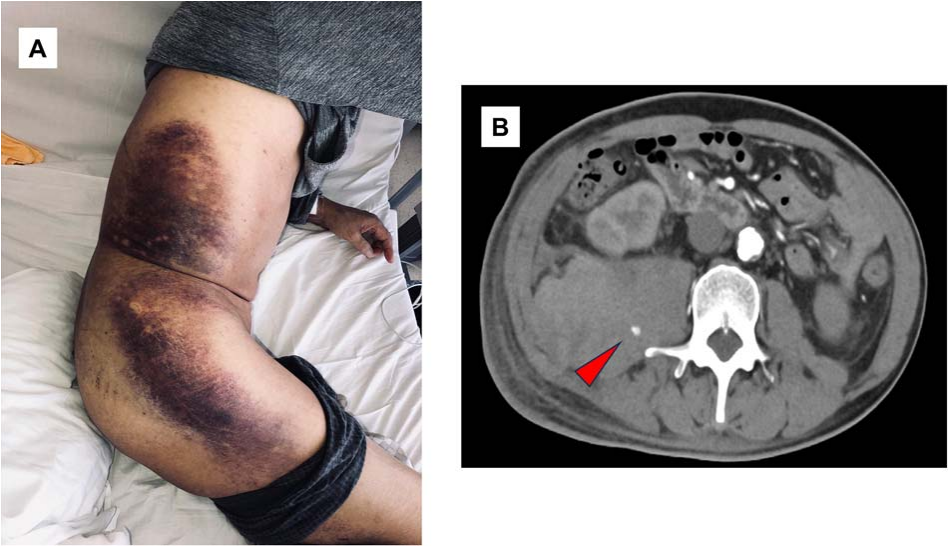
(A) Clinical photograph obtained on hospital day 8. Subcutaneous hemorrhage on the right flank and buttock. (B) Contrast-enhanced computed tomography scan obtained. Axial computed tomography scan showing right-sided retroperitoneal hematoma and lumbar artery rupture with extravasation of contrast medium (arrowhead).
